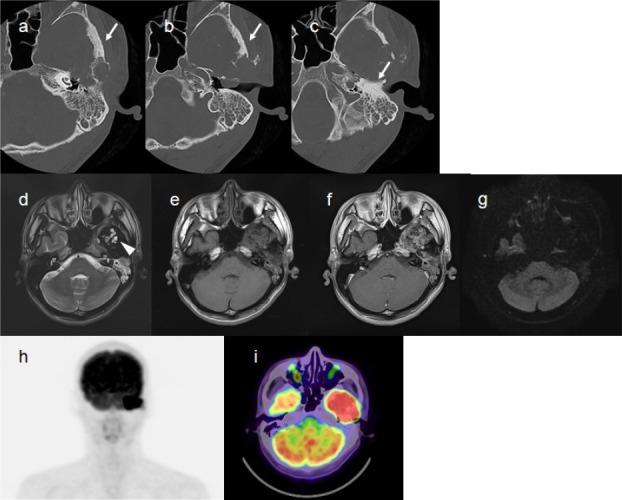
Imaging findings of case number 1. Bone-window CT images (a-c) show temporal bone defects and sclerotic changes (white arrows) around the tumor. T2-weighted MRI image (d) shows low signal intensity in most parts, with some areas of high signal intensity (white arrowhead). Plain T1-weighted image (e) and gadolinium contrast-enhanced T1-weighted image (f) show non-uniform enhancement. No elevated signal intensity is noted on the diffusion-weighted image (g). ^18^F-FDG PET/CT (h, maximum-intensity-projection image; i, fused PET/CT image at the delayed phase) shows markedly elevated uptake in the tumor. SUV_max_ values in the early phase (1h) and the delayed phase (2h) were 17.1 and 20.2, respectively; the RI was 18.1%
